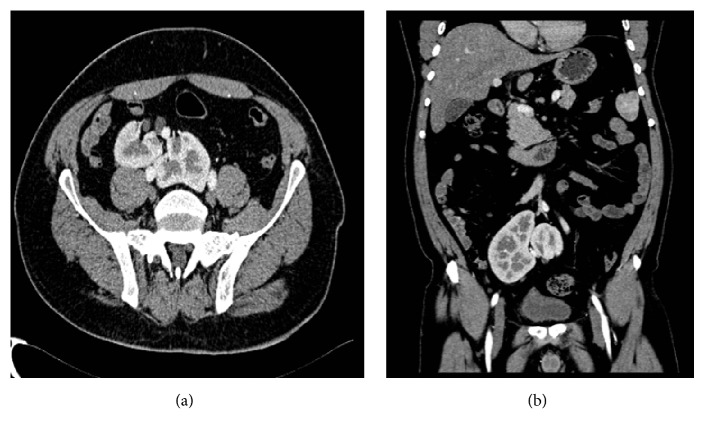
Axial (a) and coronal (b) CT scan, with intravenous contrast in the corticomedullary phase, showing a clear plane of separation between the two kidneys.
